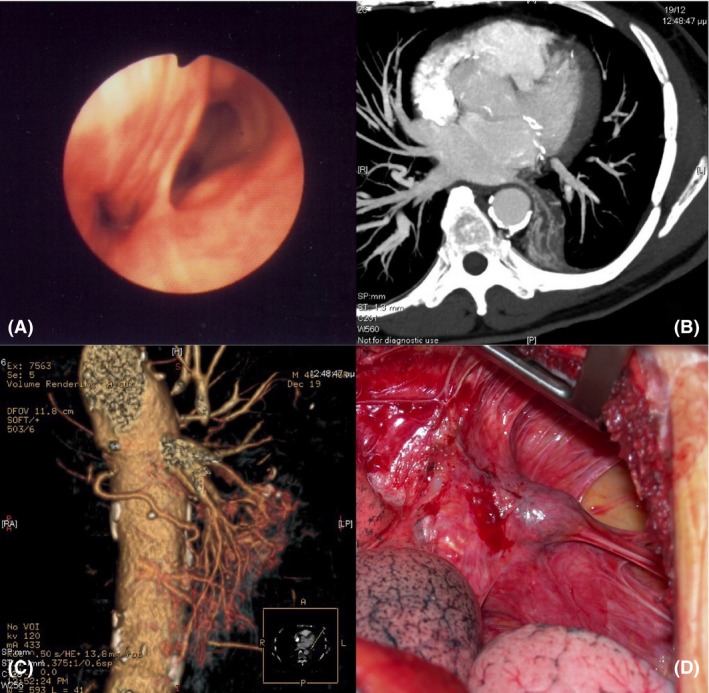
(A) Bronchoscopy reveals an ectopic port of the left main bronchus. (B) Chest computed tomography image shows ectopic perfusion of the lesion from the aorta. (C) Three‐dimensional maximum intensity projection computed tomography image shows ectopic perfusion of the lesion from the aorta. (D) The lesion during left posterolateral thoracotomy.
